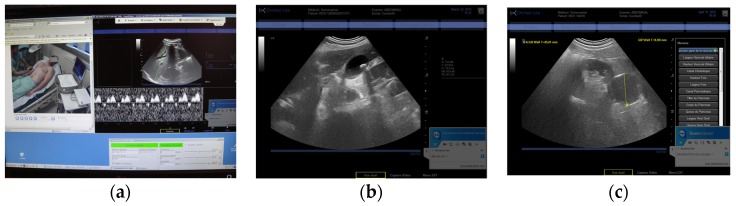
(a) Expert center computer screen for the MP with video of the patient (left), hepatic vein Doppler (right), and probe engine interface (bottom right); (b) View of gallbladder with lithiasis inside; (c) View of a renal cyst.
